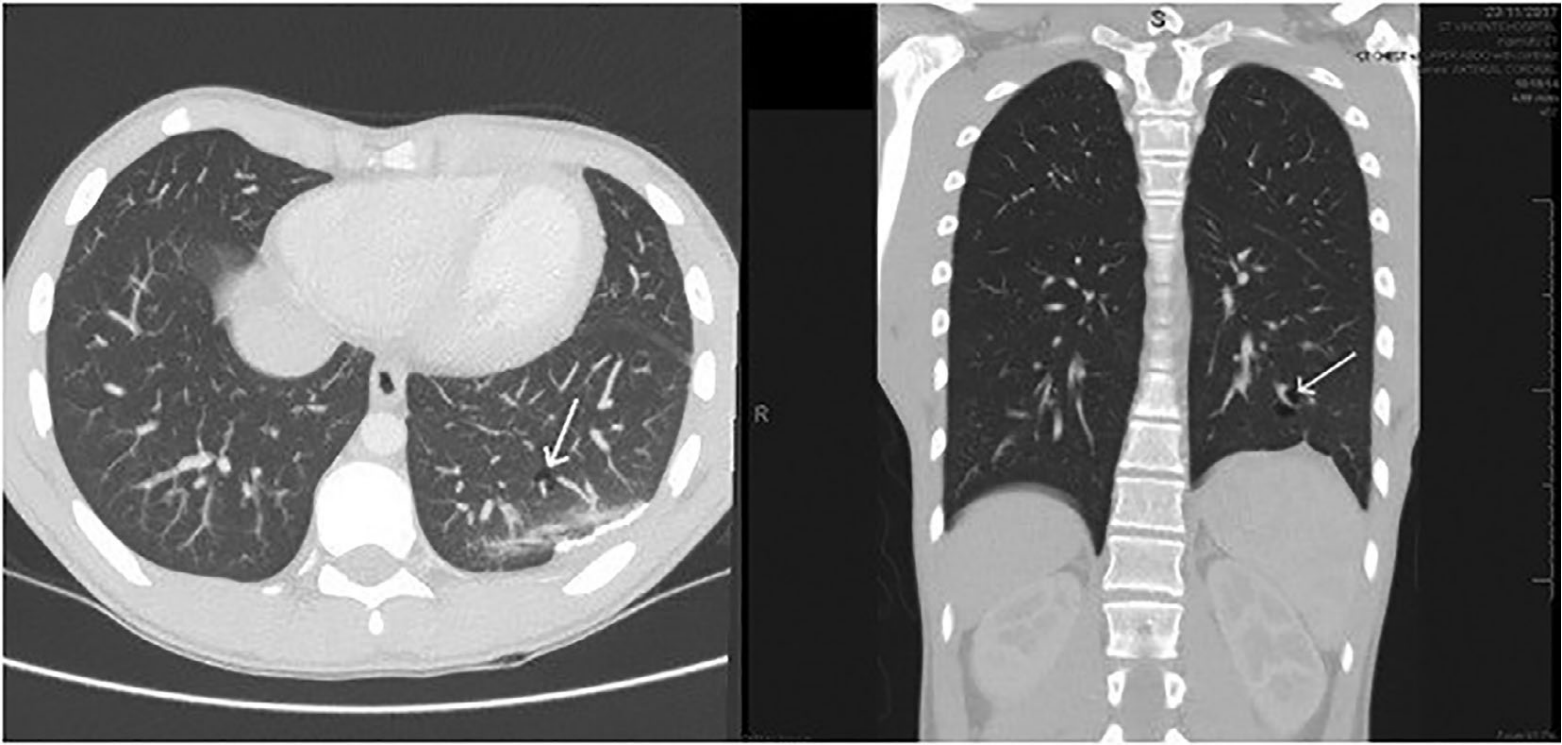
High‐resolution computerized tomography (HRCT) of the chest demonstrating multiple, small, subpleural parenchymal lung cysts.
